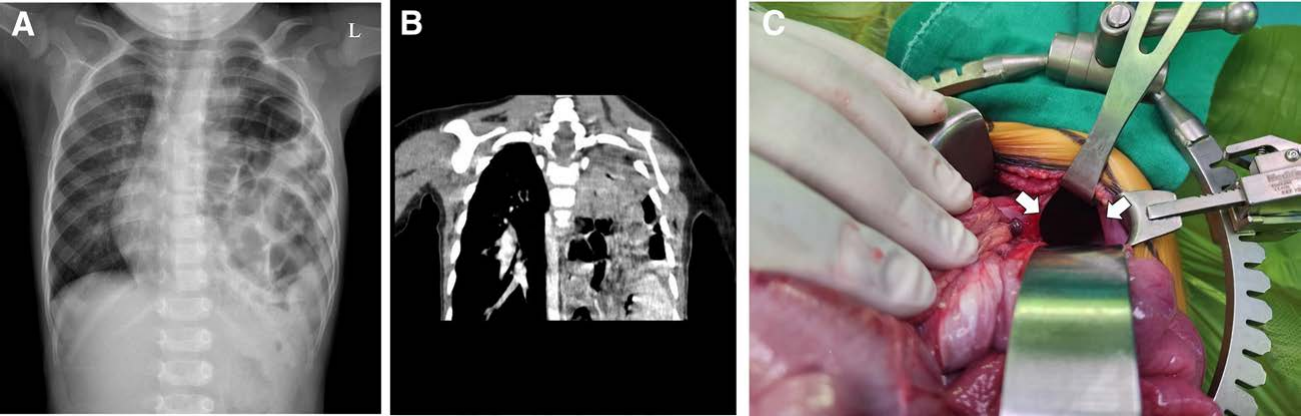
Preoperative imaging and intraoperative findings in case 4. (A) Chest X-ray and (B) CT scan reveal multiple herniated bowel loops in the left thoracic cavity. (C) Intraoperative findings show a diaphragmatic defect (arrow) after the reduction of the herniated organs. CT = computed tomography.
